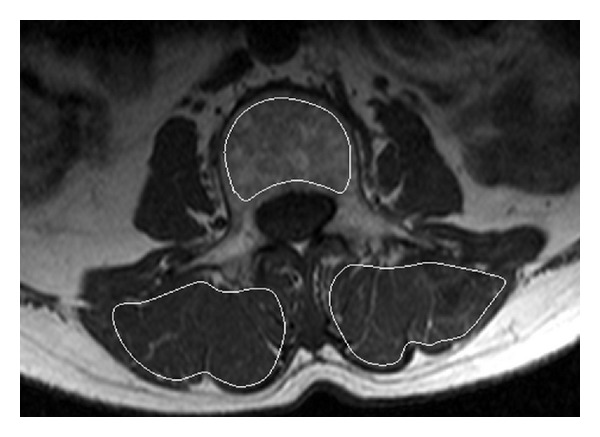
T1-weighted MR image in axial plane (from a subject with normal BMD). The image shows manually drawn ROI positioned within cortical margins of L3 vertebral body and erector spinae muscle for time-signal intensity data points measured from dynamic contrast enhanced images.
